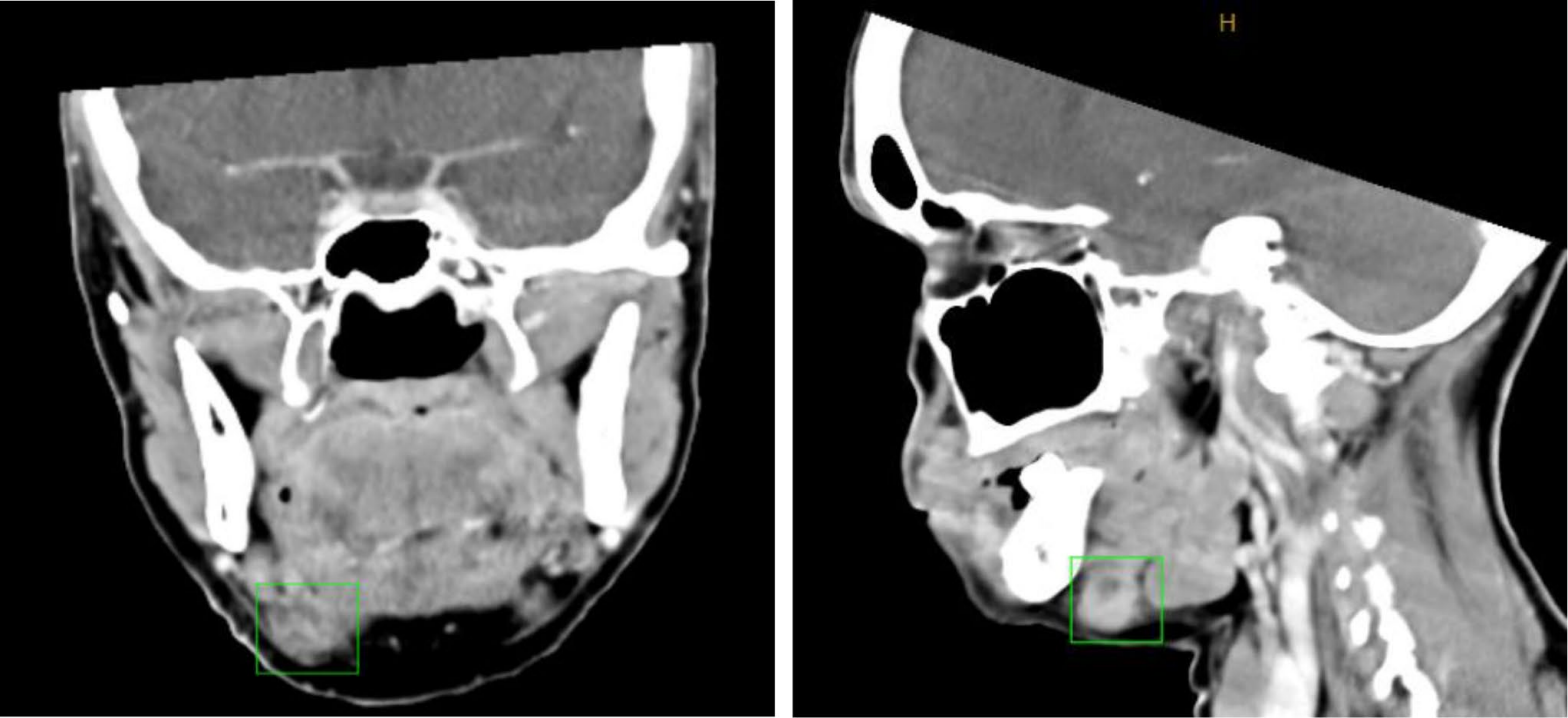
CT scan demonstrating enlarged right submandibular lymph node with necrotic appearance
